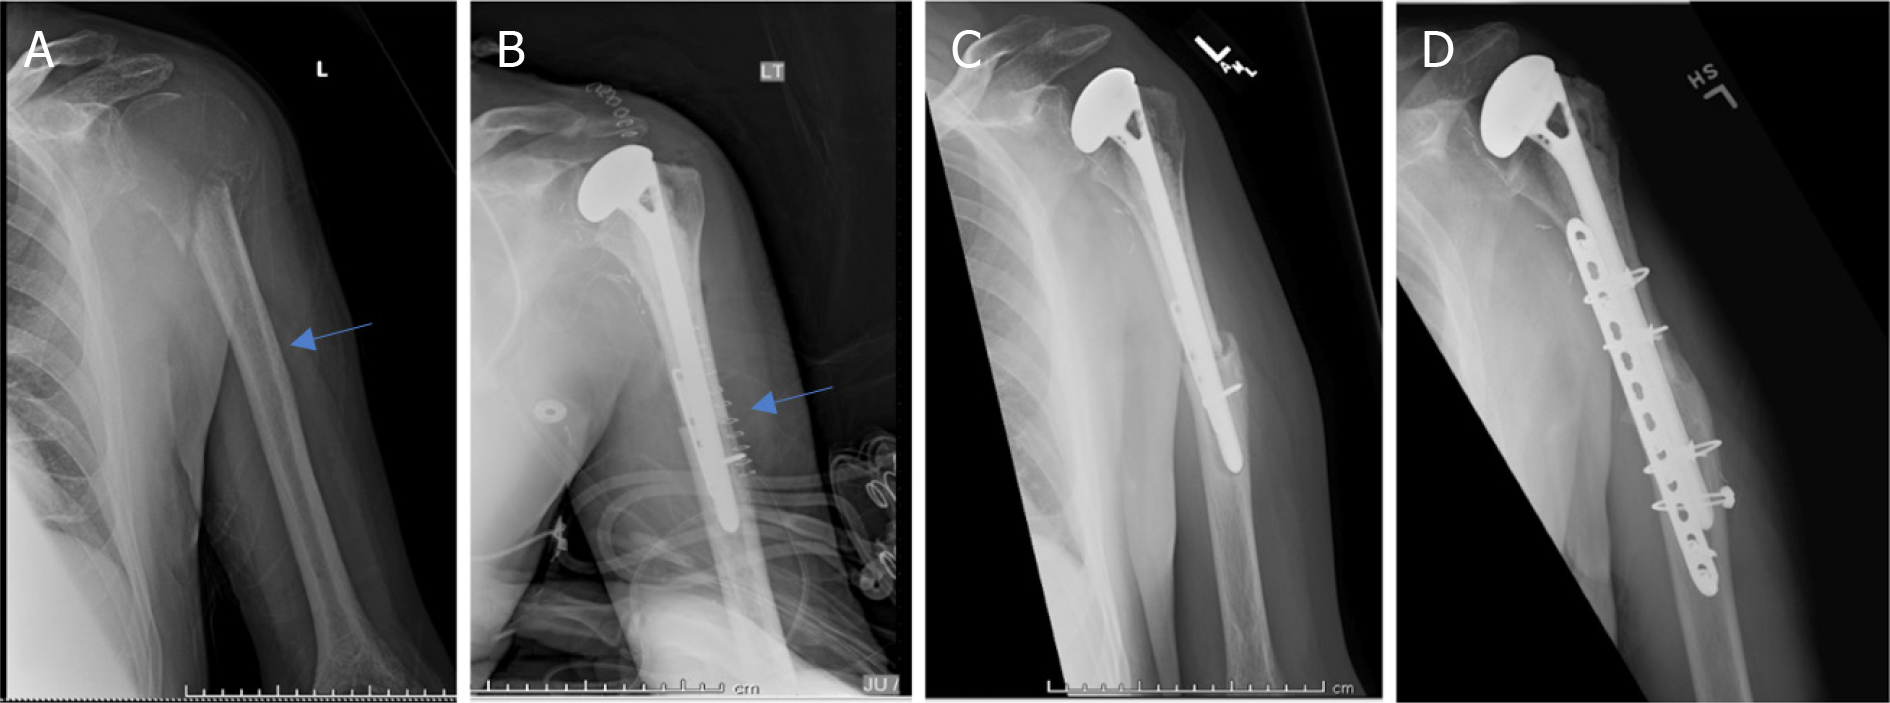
Anteroposterior radiographs of a 55-year-old male with: giant cell tumor of the proximal humerus preoperatively (A); six months after wide excision and proximal humeral APC reconstruction (B); patient was noted to have an allograft-host non-union with hardware failure at the two-year follow-up visit (C); anteroposterior radiographs after bone grafting, repair of the non-union with open reduction and internal fixation (D) Blue arrow notes that junction between allograft and native bone.
